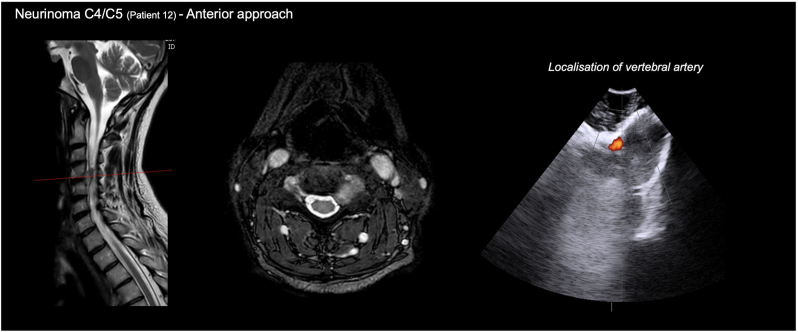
Augmentive Application of intraoperative Ultrasound. Representative case of a 57-year-old female patient with a C4/C5 neurinoma treated via an anterior approach. The left and middle panels show preoperative T2-weighted MRI in sagittal and axial views. The right panel displays the intraoperative ultrasound image, which enabled visualization of the vertebral artery to ensure its preservation during tumor resection.
